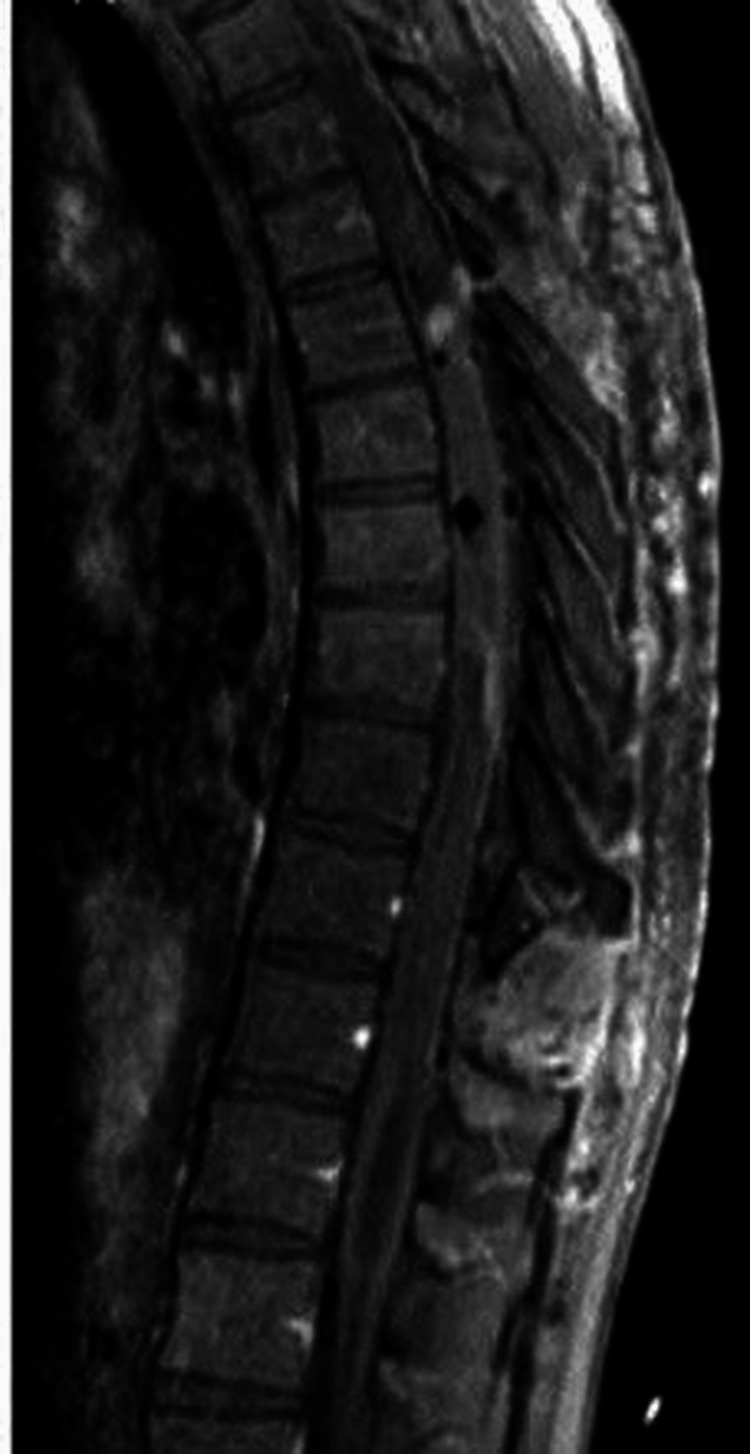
Postoperative MRI of the thoracic spine showing complete removal of the lesion Postoperative T1-weighted sagittal MRI of the thoracic spine with gadolinium enhancement showing that the lesion was gross-totally excised.
